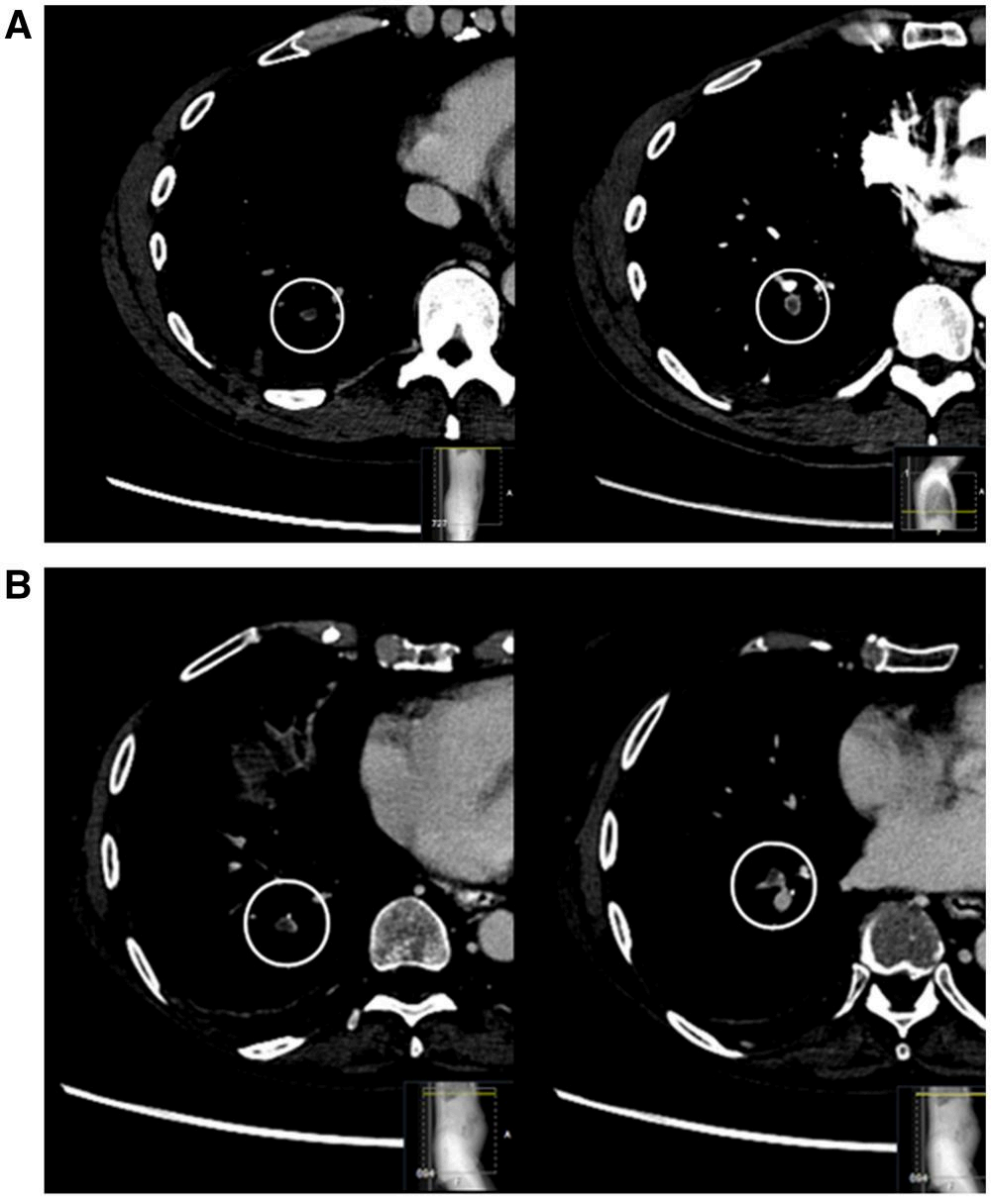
Cases of reported iPE that were AI false negative in standard contrast-enhanced abdominal CT. (A) A middle-aged man with upper right quadrant abdominal pain and elevated C-reactive protein levels, AI false negative for PE. A filling defect in a subsegmental artery in the right lower lobe was reported as suspicion of PE, shown to the left. Confirmatory CTPA was recommended, showing that the PE in the right lower lobe was segmental (shown to the right), with additional PE in the middle lobe and the left lung (not shown). (B) An older women with pancreatic cancer and metastases, presenting with abdominal distension and nausea. AI false negative for PE. Abdominal CT shows tumor progression and PE in segmental and subsegmental arteries, visualized in the axial plane. AI = artificial intelligence; CTPA = CT pulmonary angiography; PE = pulmonary embolism.
